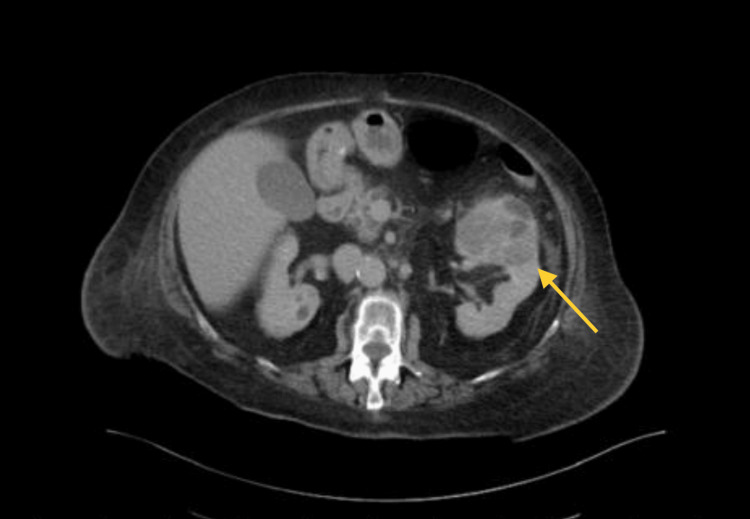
A computed tomography scan showed a left kidney mass (arrow) with features suggestive of primary renal cell carcinoma
